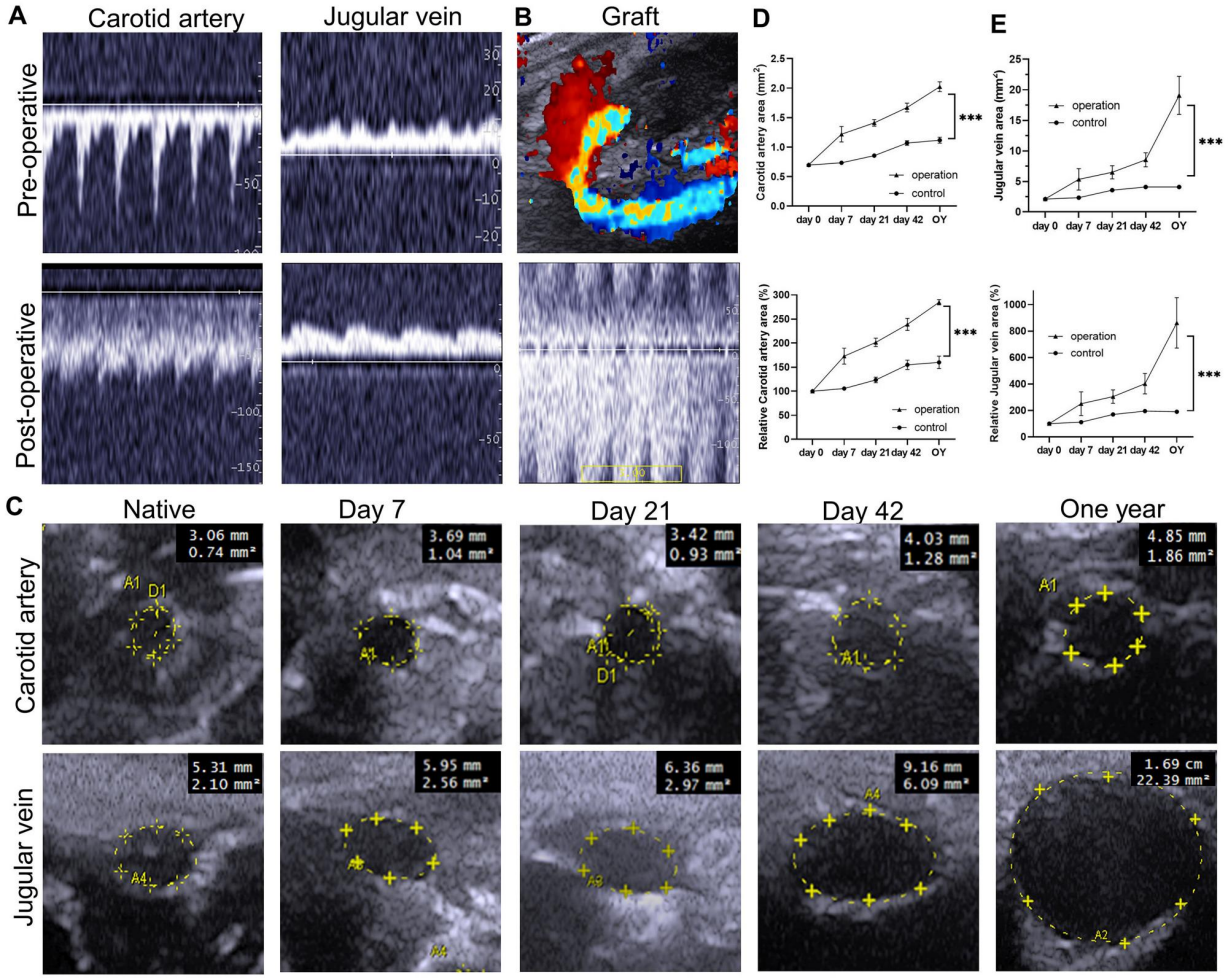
Assessment of decellularized vascular grafts *in vivo* using non-invasive ultrasound. (A) Ultrasound spectral analysis of the carotid artery and jugular vein before and after transplantation of decellularized vascular grafts. The appearance of AVG-specific spectral patterns in the carotid artery and jugular vein after transplantation indicates successful modeling. (B) Color Doppler blood flow imaging and ultrasound spectrum of decellularized vascular grafts. (C) Evaluation of changes in the lumen size of the carotid artery and jugular vein. (D) Statistical analysis of the area and relative area of the carotid artery after transplantation (*P* < 0.0001, ANOVA; Day 0, Day 7, Day 21 and Day 42, *n* = 5; 1 year, *n* = 3). (E) Statistical analysis of the area and relative area of the jugular vein after transplantation (*P* < 0.0001, ANOVA; Day 0, Day 7, Day 21, and Day 42, *n* = 5; 1 year, *n* = 3).
